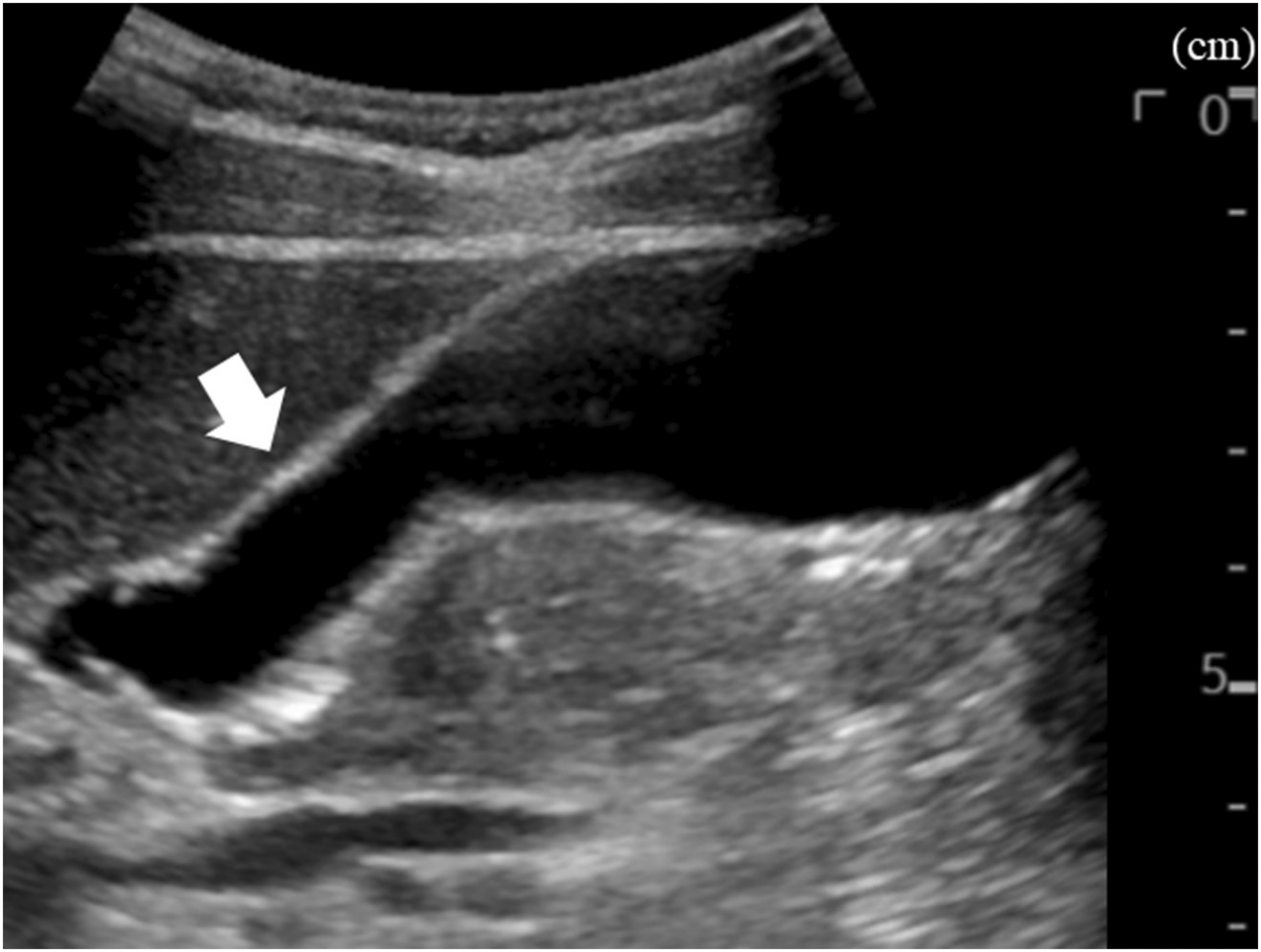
Findings of the gallbladder wall on abdominal ultrasonography 6 days after discharge. Abdominal ultrasonography 6 days after discharge showing improved gallbladder wall thickening, with a measurement of 1.5 mm (arrow).
